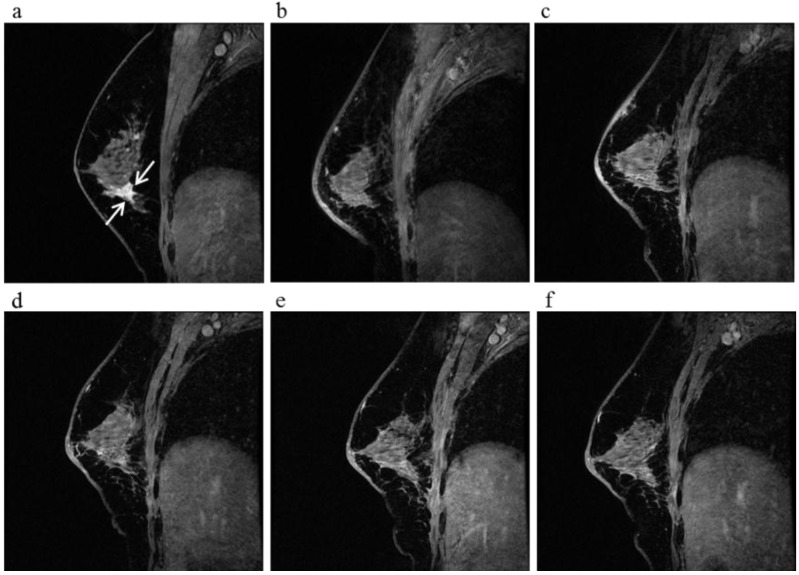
A 60-year-old female (case 4 in Table 1, Table 2 and Table 3) with right breast cancer (cT1cN0M0). CE breast MRI (a) revealed a breast tumor before KORTUC II therapy, the region of tumor was 18 mm (arrows); After KORTUC II, no recurrence was identified on CE breast MRI ((b): 5 month; (c): 17 month; (d): 29 month; (e): 41 months; (f): 53 months).
